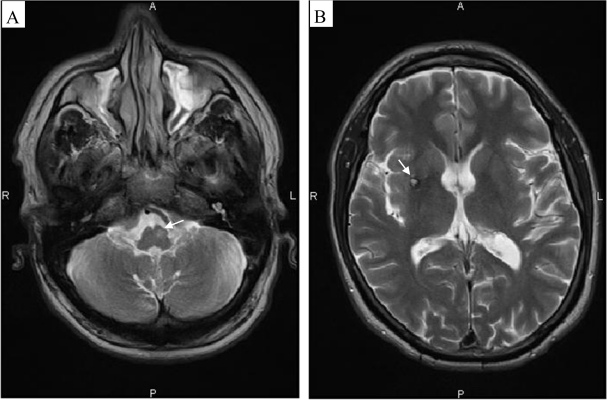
Brain MRI of a patient exhibiting a disabling neurological tremor in the upper limbs. Note the left inferior olivary hypertrophy (Guillain-Mollaret triangle; arrow in left panel, (A) and the lesion in putamen (arrow in right panel, (B). Axial T2-weighted images. R: right, L: left.
